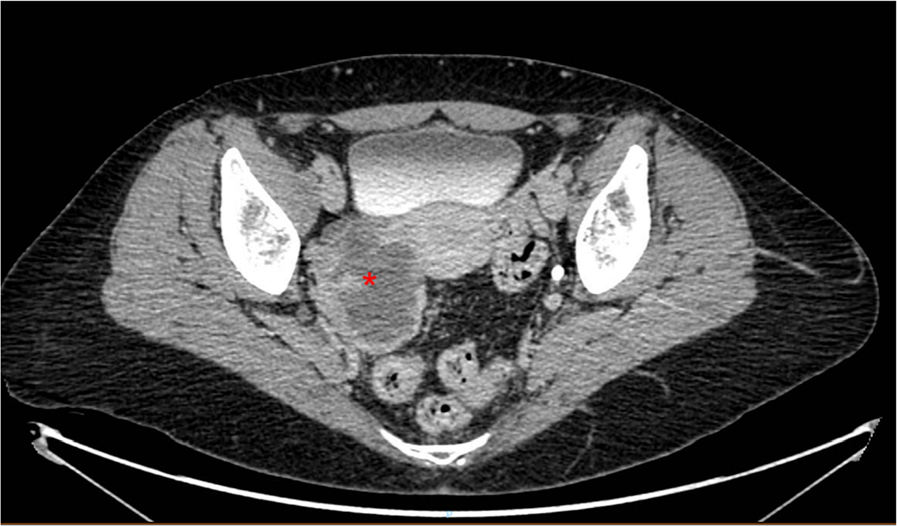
CT scan image. The single star shows the pelvic mass
